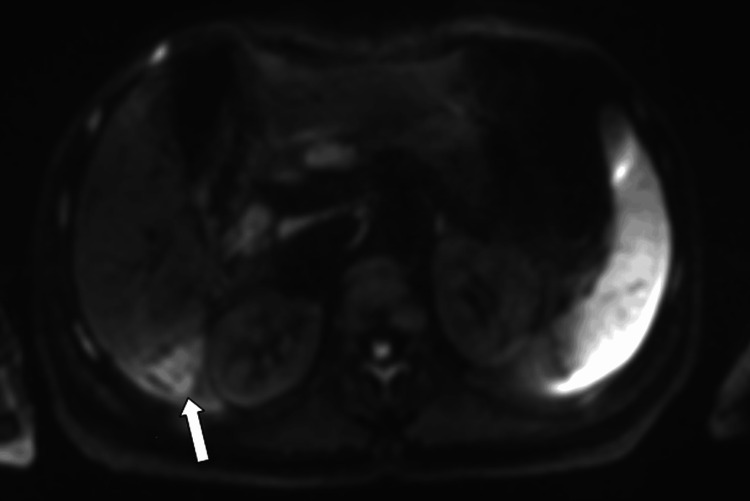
Axial diffusion-weighted MRI (b-value 800) image at the level of the lesion Axial diffusion-weighted MRI (b-value 800) demonstrating hyperintensity of the intraductal lesion in segment VI, consistent with restricted diffusion (white arrow).
